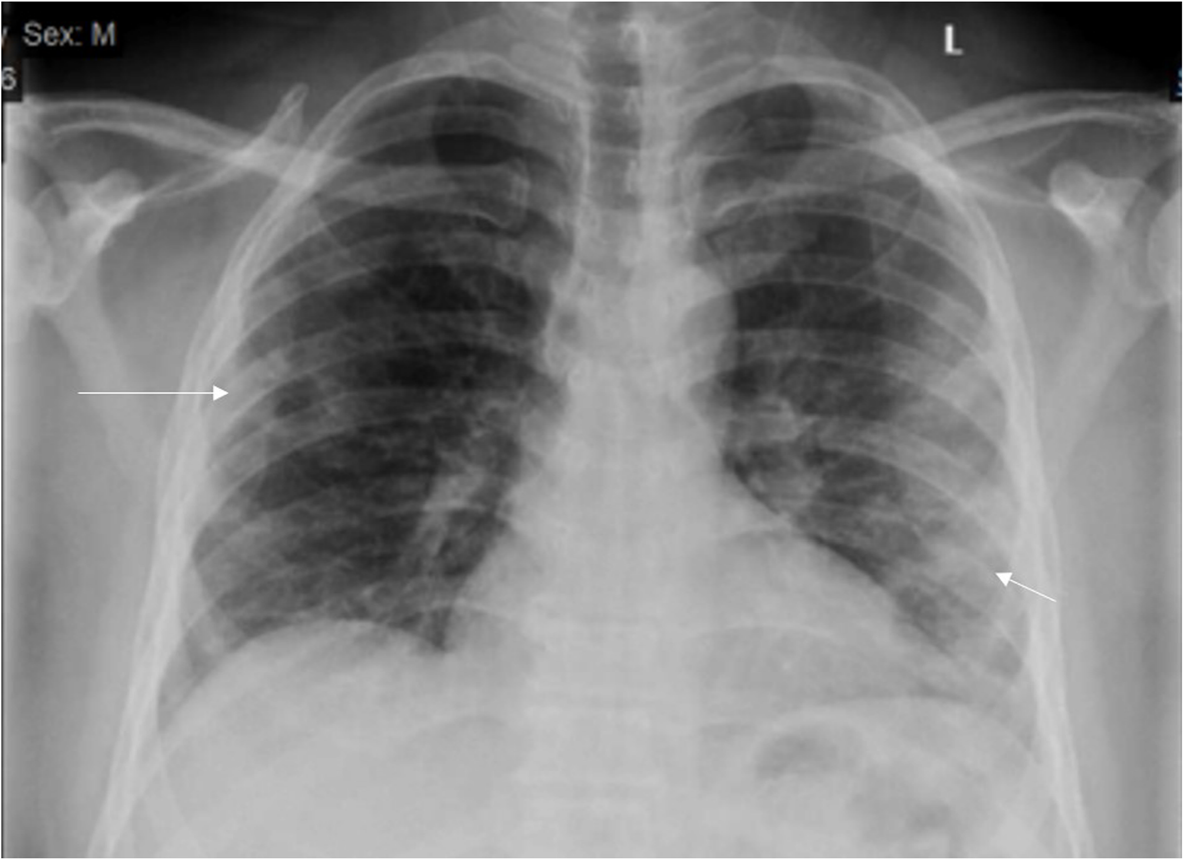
54-year male patient with positive COVID-19. He was complaining of cough and fever. Follow-up chest X-ray showed right midzonal lesion with central lucency giving atoll sign (long arrow) of organizing pneumonia. Also, left lower zonal small air space consolidation opacities with reticular thickening was seen (short arrow). The severity score was 1 for each lung, so the total severity score was 2
